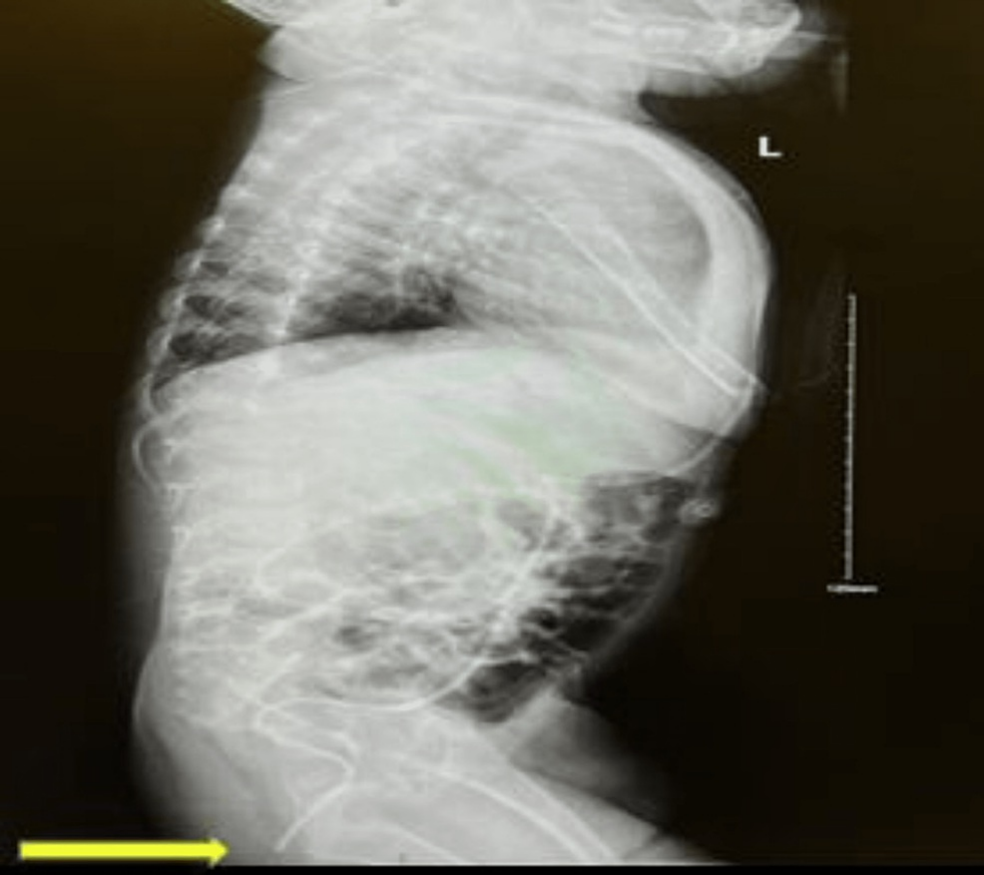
The VP shunt survey X-ray. The image is confirmed the peritoneal end of the shunt tube is going well beyond the pubic symphysis.
